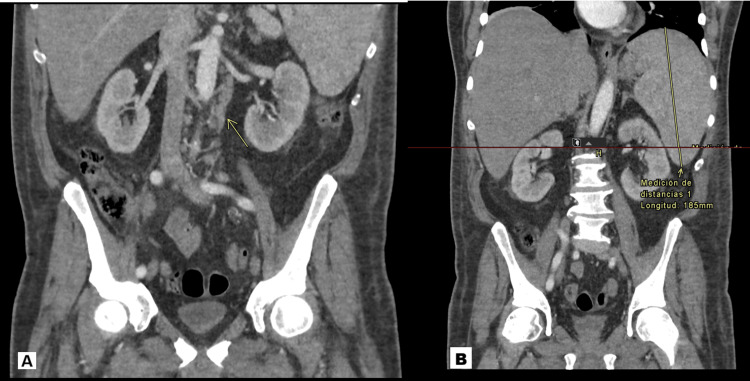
Contrast-enhanced thoracoabdominopelvic computed tomography (CT) A) Extensive infiltrative para-aortic lymphadenopathy, forming nodal conglomerates that suggest vascular encasement or displacement. B) Significant splenomegaly (18.5cm)  with a multinodular pattern, showing multiple poorly defined, diffuse hypodense lesions throughout the splenic parenchyma.
